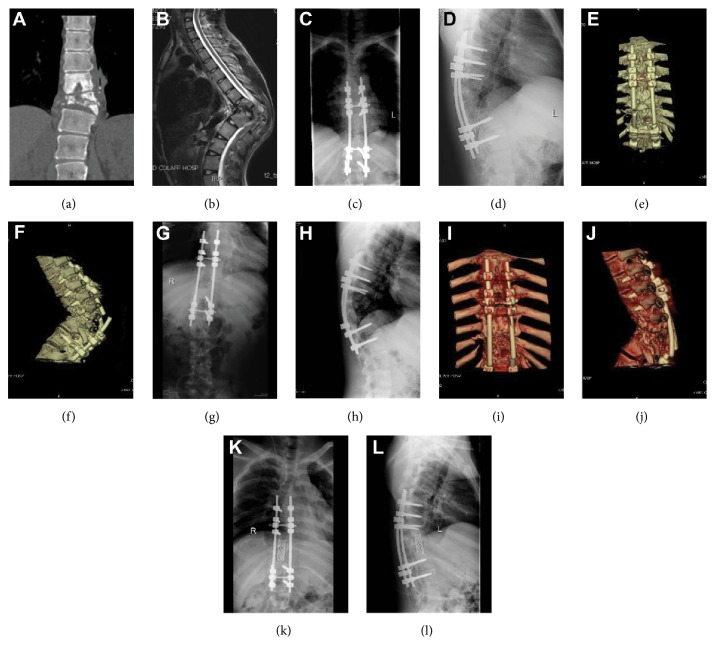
(a)-(b) Preoperative imaging study of an 18-year-old male showing thoracic vertebral tuberculosis and thoracic kyphosis. (c)-(d) The posterior approach was used for surgery, and postoperative imaging data show satisfactory positioning of the internal fixation device. (e)-(f) Three-dimensional reconstruction of CT scan images showing breakage of the internal fixation device after surgery. (g)-(h) The posterior approach was used for the initial repair surgery. The anteroposterior and lateral radiographic views show satisfactory positioning of the internal fixation device after surgery. (i)-(j) Three-dimensional reconstruction of CT images showing breakage of the internal fixation device after the initial repair surgery. (k)-(l) The combined anterior and posterior approach was used for the secondary repair surgery. Anteroposterior and lateral radiographic views show satisfactory positioning of the internal fixation device. The lesion has been completely removed.
